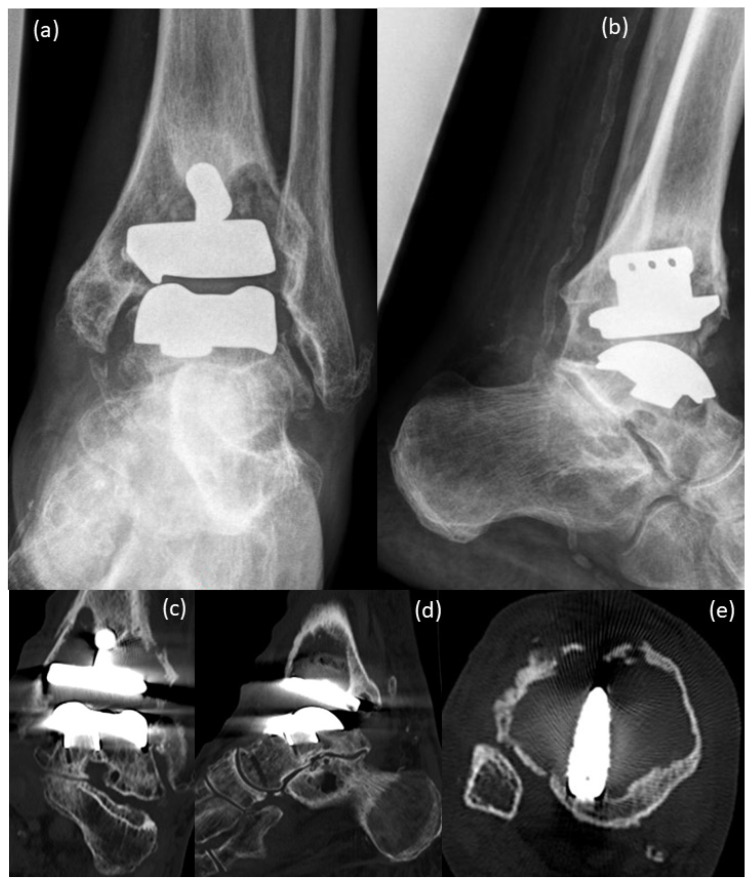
Pre-surgical images 2020: (a,b) X-rays, (c–e) CT scans of the patient; (a,b) large cysts are present in the tibia and in the talus, compared to the images from the year 2016 (Figure 1), a massive increase in bone resorption and cyst formation is seen around the tibial component under involvement of the stem of the prosthesis. Therefore, an increasing tilt of the joint surface of the tibial component can be seen as a sign of incipient dislocation; (c–e) CT scans confirm the X-ray findings with massive bone resorption, pathologic fracture of the lateral tibial cortical surface and instability of the tibial prosthesis component.
